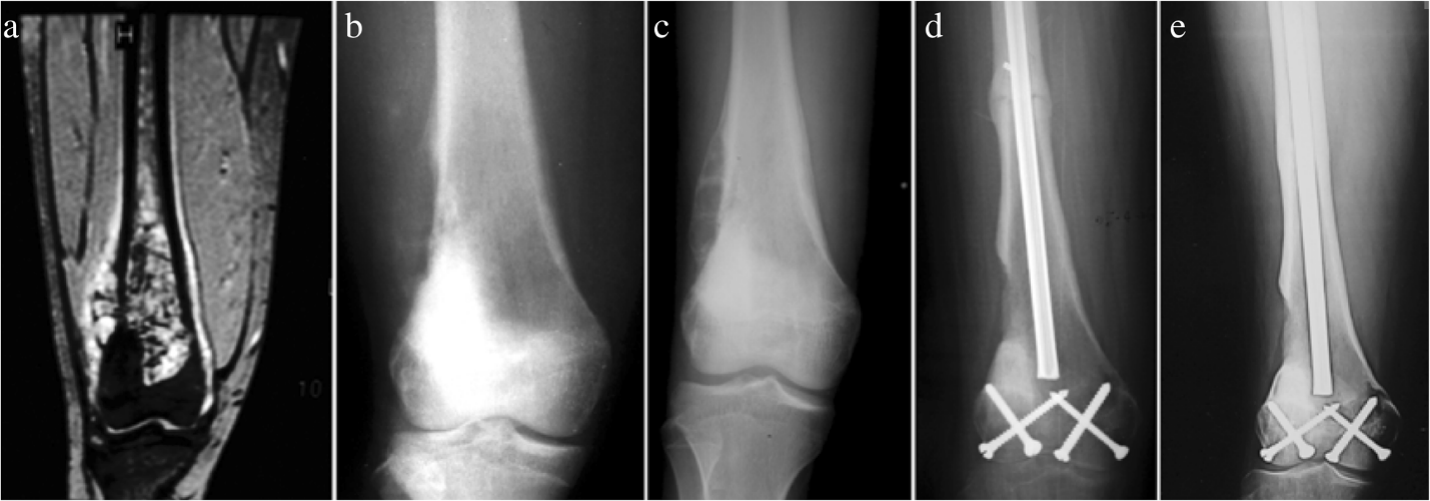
A 15-year-old female patient with osteosarcoma in right distal femur was treated with alcohol-inactivated autograft replantation with articulation preservation. a. Preoperative MRI showed intramedullary high and low mixed signal in distal femur. The tumor lies near Insall line which was classified as Type II. b. Preoperative X-ray showed osteolytic bone destruction in distal femur, in accompany with severe bone destruction in lateral cortical bone, local soft tissue mass and periosteal reaction. c. Postoperative X-ray showed significantly reduced osteolytic bone destruction in distal femur, in accompany with local soft tissue mass disappearance and restoration of the continuity of the lateral periosteum. d. Two months after operation, X-ray showed bone callus in diaphysis, and bony healing in conjunction between host bone and inactivated bone. e. 110 months after operation, X-ray showed fully bony healing and good joint space.
